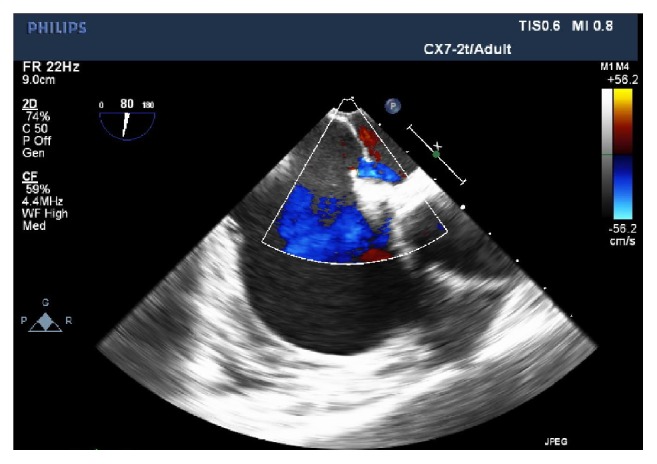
TEE mid-esophageal RV inflow-outflow view with color flow Doppler showing atria septum consistent with PFO (predominant R-to-L shunting).
